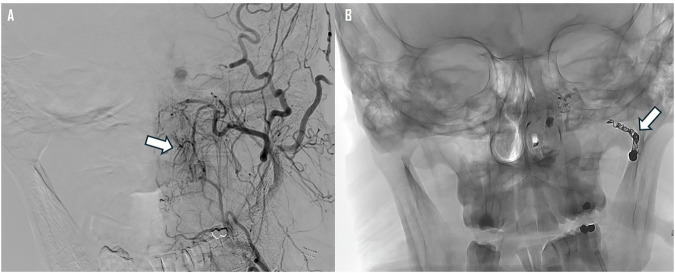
Angiographic images of epistaxis embolization. 47-year-old male patient status post renal transplant. Had left-sided epistaxis on day 1 post-op. Front view angiographic image with subtraction (A) revealing a contrast blush from the left maxillary artery. Dense material in the embolized area (B) corresponds to the radiopaque component of the tamponade. The primary embolization was performed using liquid embolic agents, specifically NBCA (n-butyl-cyanoacrylate) combined with lipiodol, with coiling as a second-line measure to augment occlusion of the maxillary artery. Front view conventional radiographic image confirming complete occlusion of the left maxillary artery after deploying Tornado coils (4–6 mm) into the feeding vessels
